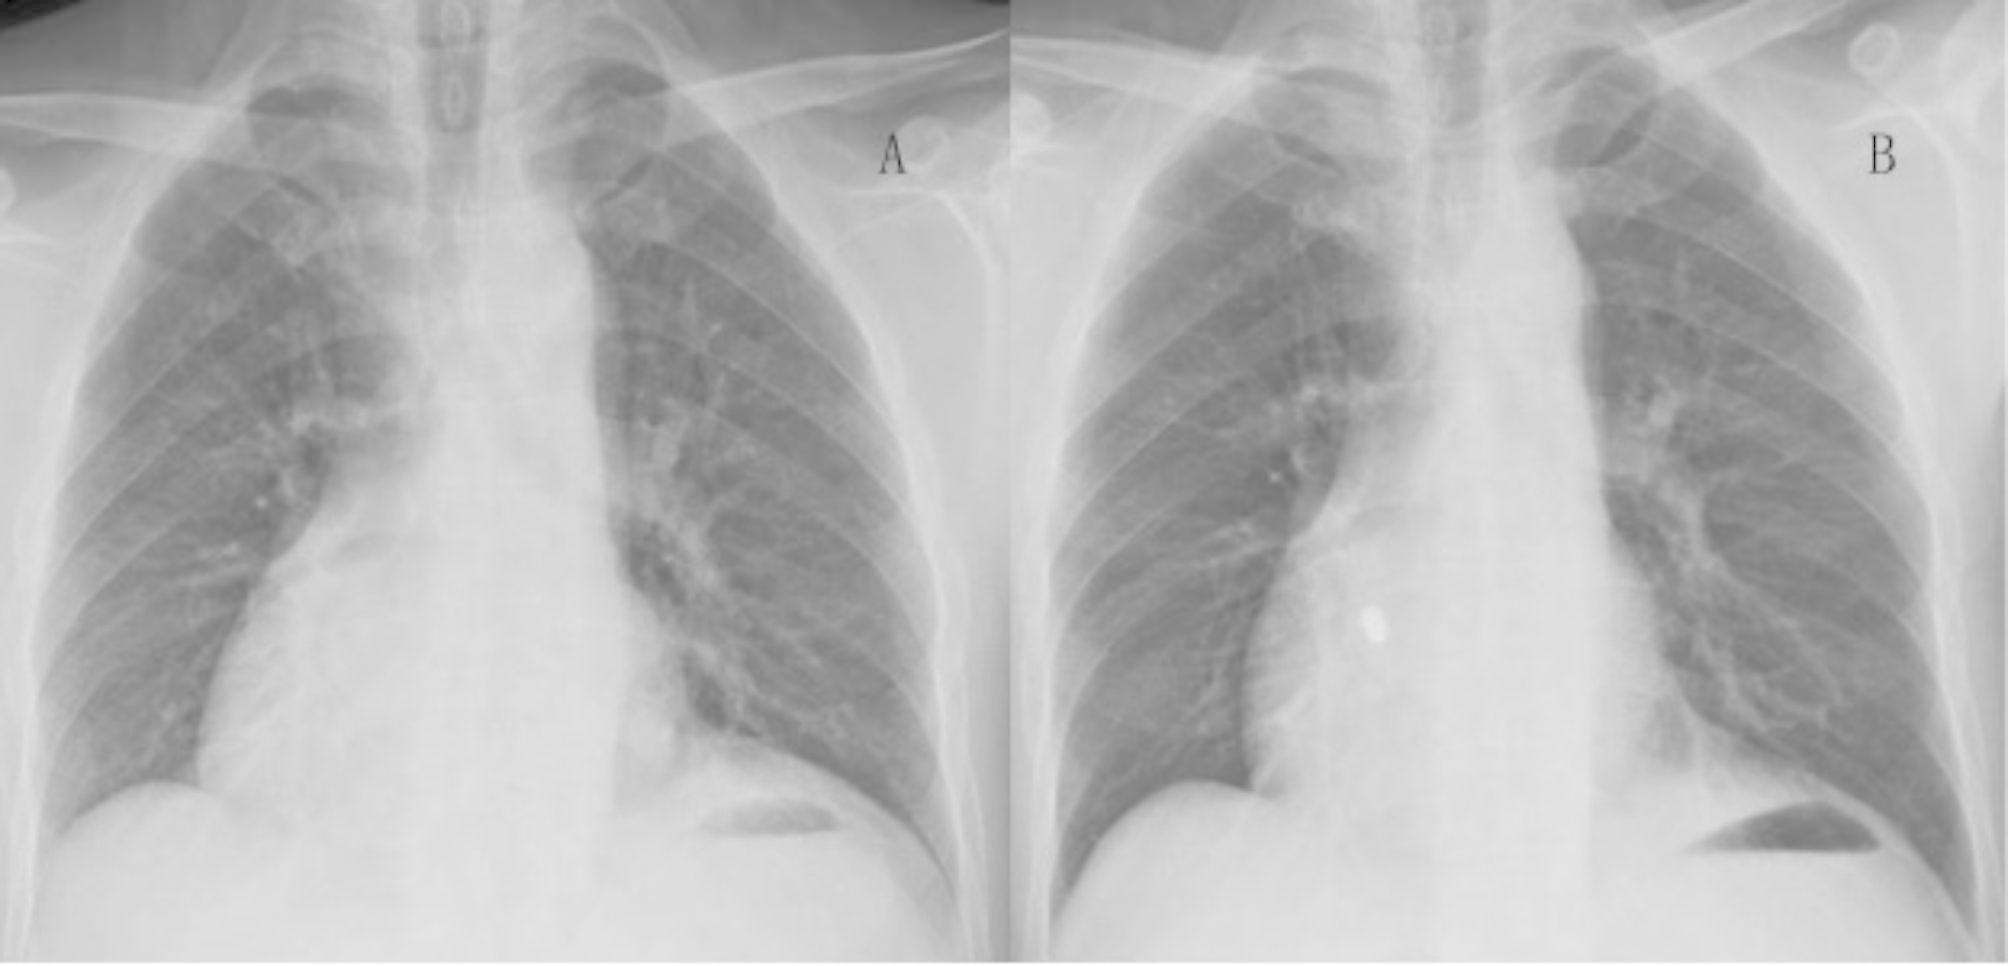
Comparison of preoperative and postoperative chest radiographs (A: preoperative chest radiograph; B: postoperative chest radiograph). The chest X-ray before discharge showed that the Micra position was fixed, and the cardiac shadow and costophrenic angle did not change significantly before and after procedure
